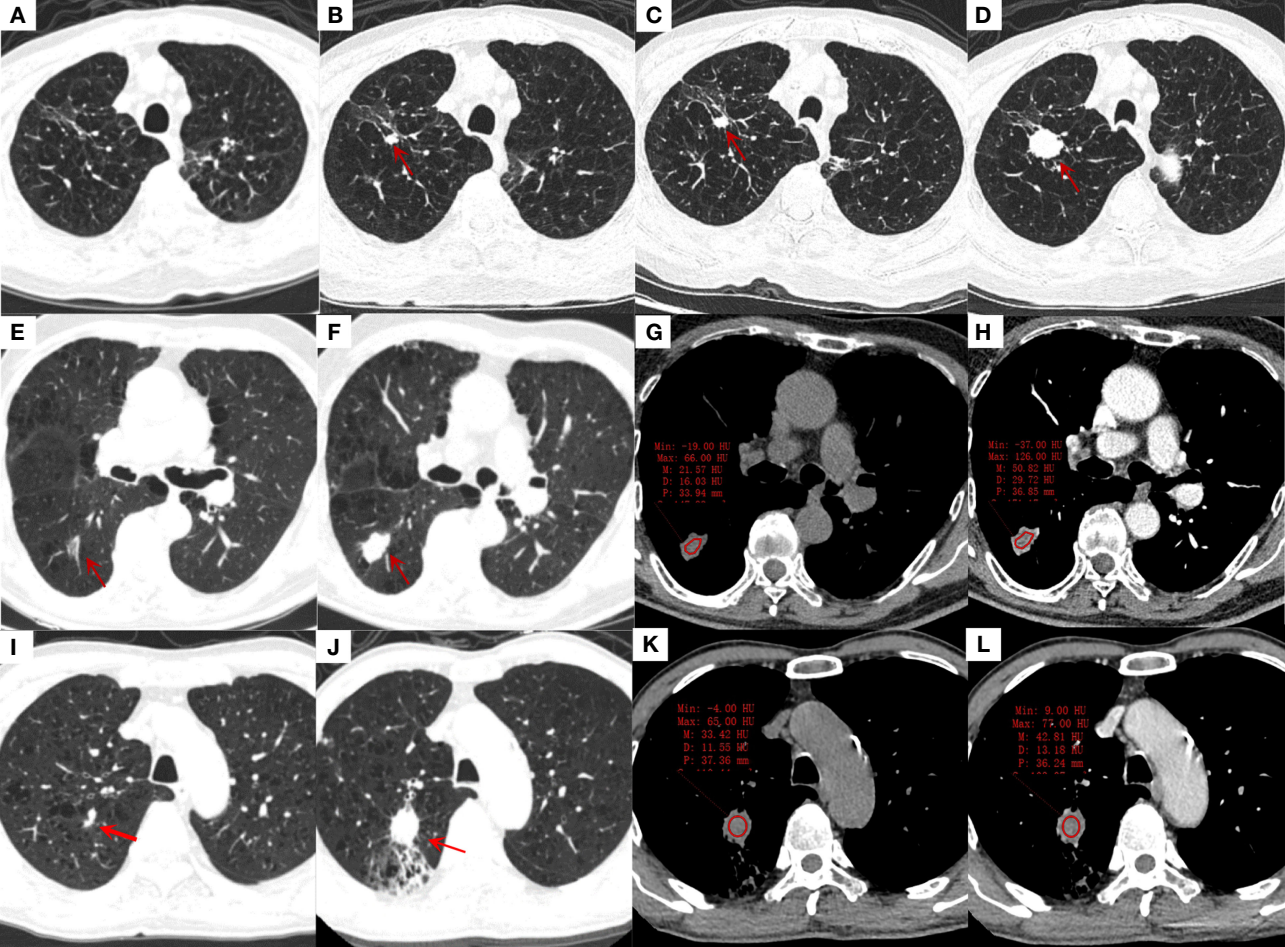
Transverse thin-section CT scans in the diagnosis of lung cancer in a lobe affected by tuberculosis. (A-D), an 85-years-old male with stable tuberculosis; (A): The first CT scan of the patient showed a few irregular linear and ground glass opacification in the right upper lung; (B): CT scan was reexamined 4 years later, showing a new solid nodule (red arrow) with a diameter of 9 mm and lobulations in the original tuberculosis focus area; (C, D): CT were reexamined 3 months and 8 months after the discovery of the nodule. The nodule was gradually enlarged, and small cell lung cancer was proved by bronchoscopic biopsy. (E-H), an 82-years-old male with stable tuberculosis; (E): a ground glass nodule (red arrow) with 16mm in length and vessel convergence was found in the dorsal segment of right lower lung on the first CT scan; (F): the ground glass nodule was enlarged to solid nodule with 23mm in length, lobulations and spiculations after 4 years, adenocarcinoma was diagnosed by surgical biopsy. (G, H): The non-enhanced and enhanced CT value of nodule was 22 HU and 51 HU, respectively; moderately heterogeneous enhancement can be seen after enhancement. (I-L), a 68-years-old male with stable tuberculosis; (I): a small solid nodule beside the vessel with 5 mm in length in the posterior segment of right upper lung was misdiagnosed as fibrous tubercle on the first CT scan; (J): the nodule was obviously enlarged with 25 mm in length and distal obstructive pneumonia after 3 years, adenocarcinoma was diagnosed by surgical biopsy. (K, L): The non-enhanced and enhanced CT value of nodule was 33 HU and 43 HU, respectively, ring-like enhancement can be seen after enhancement.
